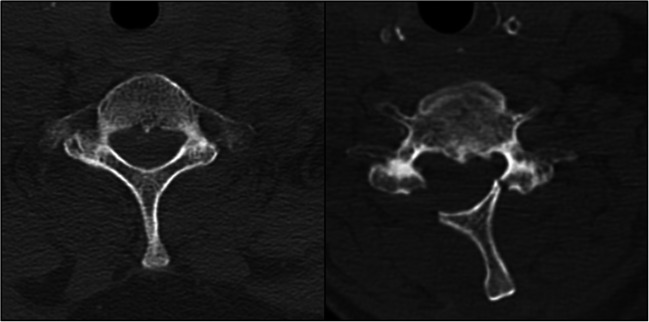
Preoperative (*left*) and postoperative (*right*) axial CT scan of a patient with cervical spondylotic myelopathy treated with laminoplasty (LP). *Right*: according to Hirabayshi’s open-door technique, the laminae are lifted on the hinges to expand the spinal canal. The spinous process was sutured to the paravertebral musculature to ensure a permanently stable position
